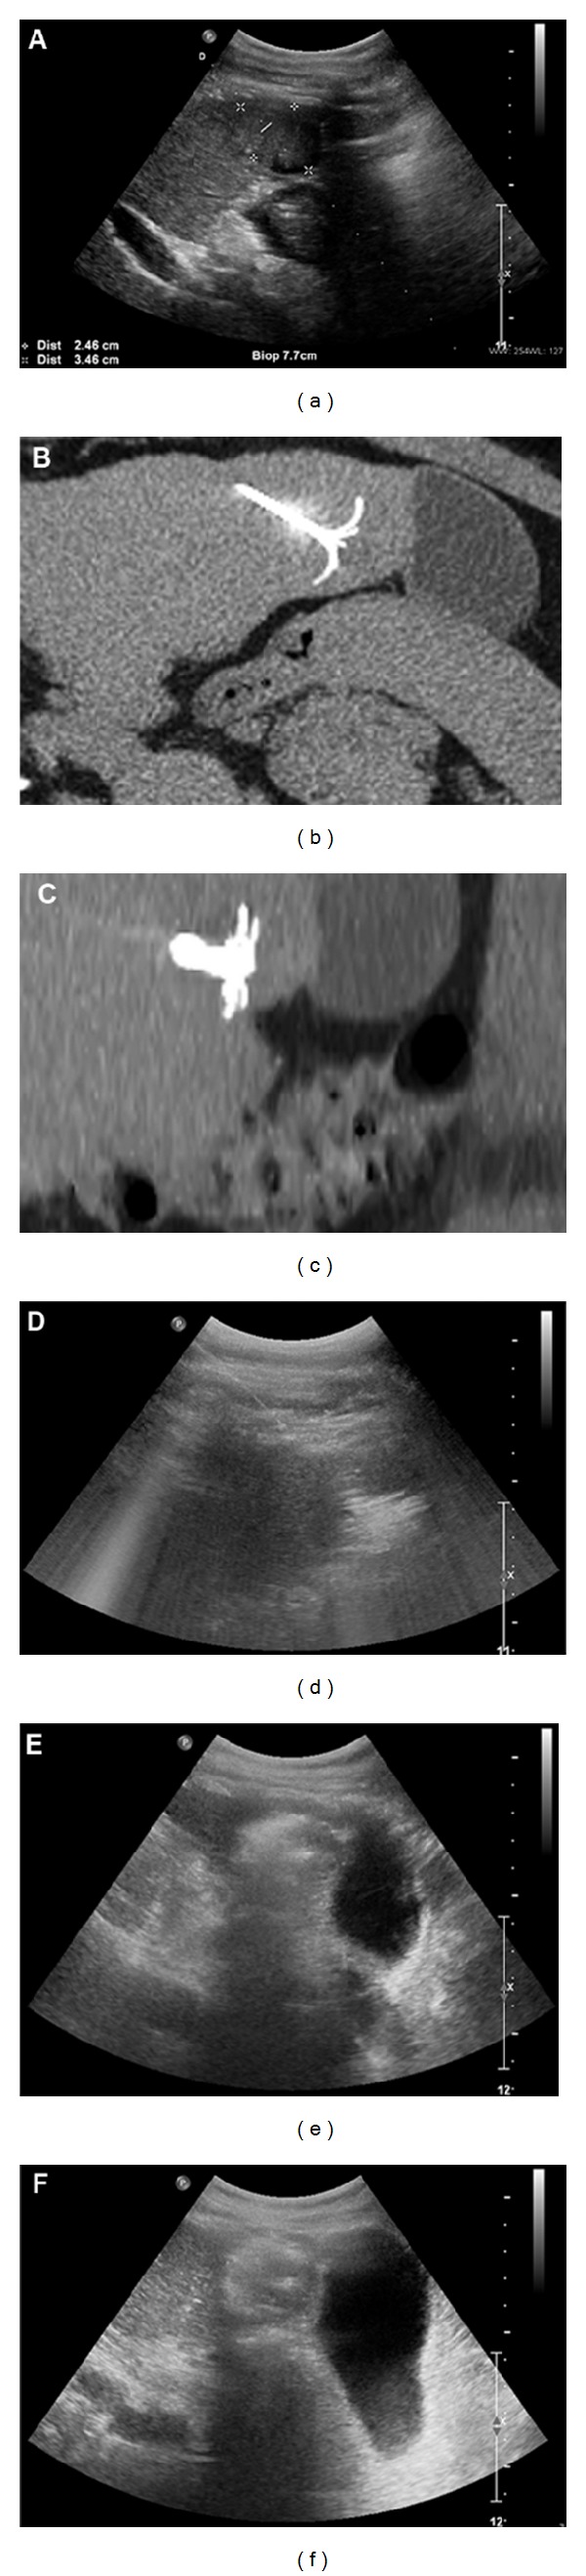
Radiofrequency ablation of the hepatocellular carcinoma performed under US and CT control with an expandable needle. (a) US view shows the hepatocellular carcinoma and the planning of the needle insertion in the liver lesion. CT axial (b) and reformatted (c) images depict the position of the needle inside the lesion. (d) US control during radiofrequency shows the hyperechoic zone that covers the entire liver lesion. US controls at the end of the first roll-off (e) and at the end of the procedure (f) do not depict any gallbladder anomalies.
